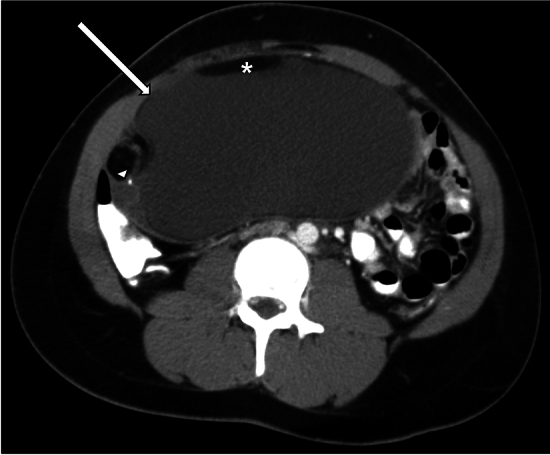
Contrast-enhanced CT of the pelvis in the same patient as Fig. 13, which demonstrates a large fluid-filled cystic structure (arrow) with adjacent solid nodule (arrowhead) containing punctate calcification. Macroscopic fat (*) is present anteriorly in the mass. Final pathology was consistent with mature teratoma. The patient’s testosterone levels normalized after surgery
